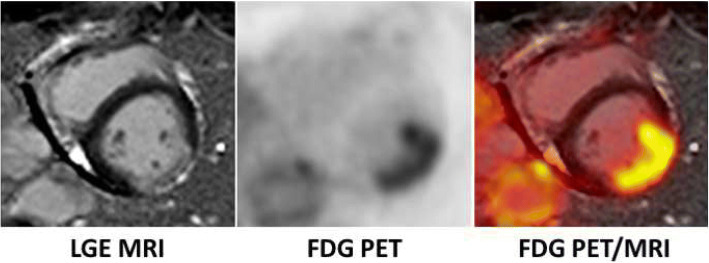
Hybrid ^18^F-FDG PET/MRI (suppressed myocardial glucose metabolism) early after ST-elevation myocardial infarction. LGE MRI (left panel) demonstrates myocardial infarction scar of the lateral wall. On FDG PET (middle panel), an intense FDG uptake matching the scar (right panel) is observed. The glucose metabolism in the scar indicates a florid inflammatory response after myocardial infarction
